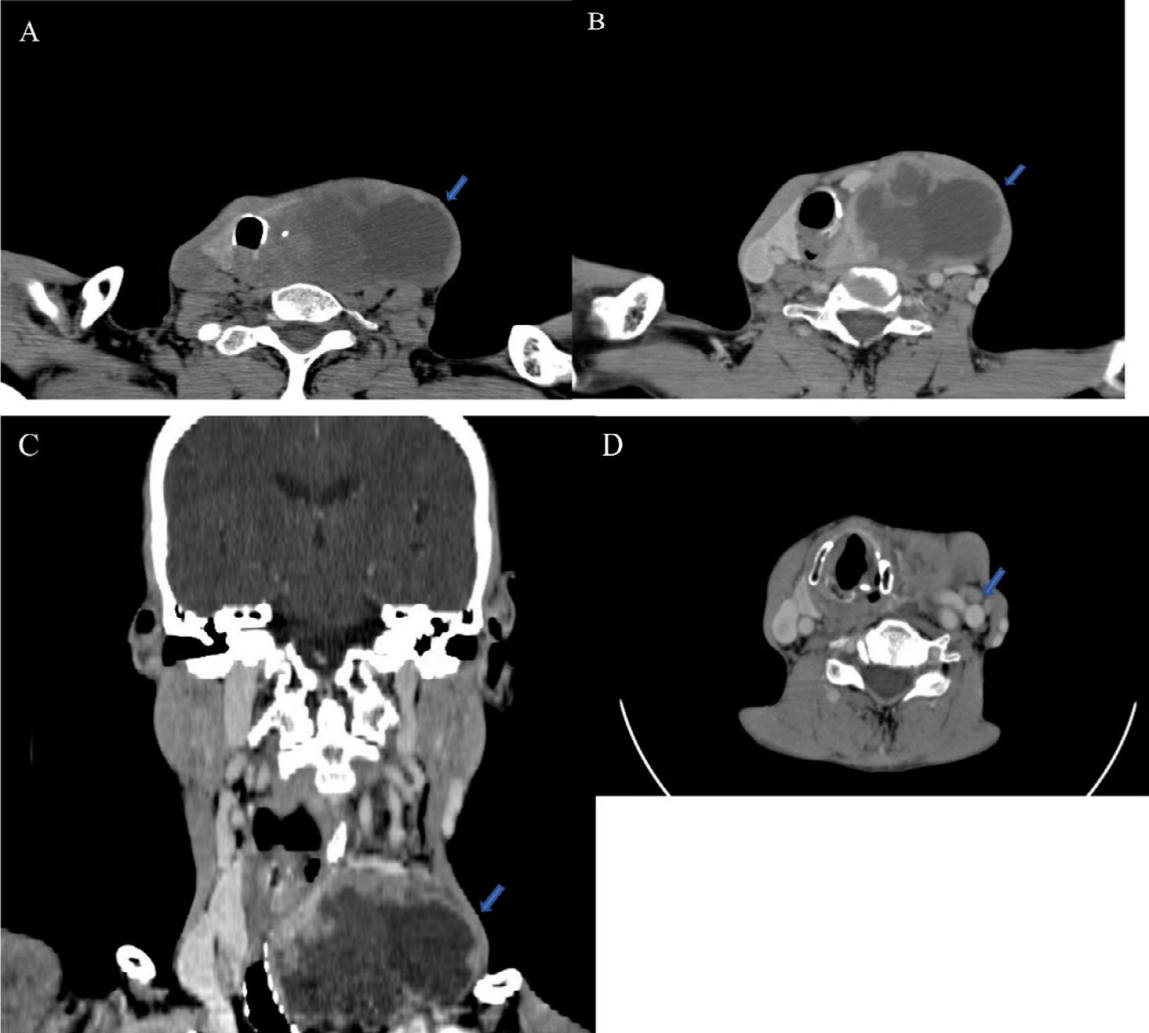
(A–D) Contrast‐enhanced CT of the neck. (A) Non‐contrast axial image showing a complex mass in the left lobe of the thyroid (arrow). (B) Post‐contrast axial image demonstrating heterogeneous enhancement of the mass (arrow). (C) Post‐contrast coronal image confirming complex enhancement (arrow). (D) Axial image showing enlarged left cervical lymphadenopathy (arrow).
